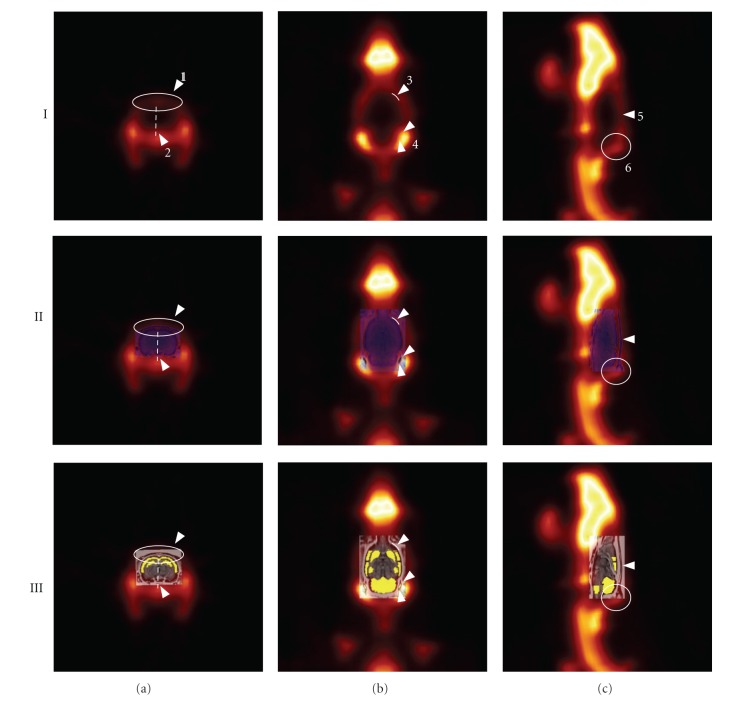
Example of an [^18^F]NaF image coregistered with MR image and the MR template. (I) [^18^F]NaF image, coregistered with (II) MR image and (III) MR template for (a) coronal, (b) transverse, and (c) sagittal cross-sections. Arrows indicate (1) medial surface cranial case, (2) ipsilateral line distributing hemispheres, (3) frontal bones, (4) tympanic bulla, (5) surface of cranial case, and (6) articulation of parietal and occipital bones.
